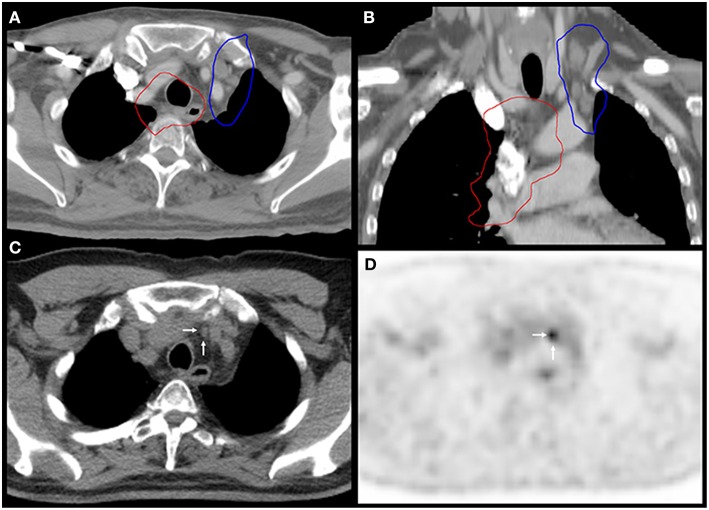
Marginal recurrence between the junctions of radiation fields. Images (A,B) show the 60 Gy isodose lines (red) from the first course of chemoradiation and the 30 Gy isodose lines (blue) from the PTV_elect_ of HIGRT-SIB on fused axial and coronal planning CT images. Images (C,D) correspond to the axial slices of the surveillance PET-CT scan that identified the marginal recurrence (white arrows) in an undertreated lymph node between the junctions of the radiation fields.
